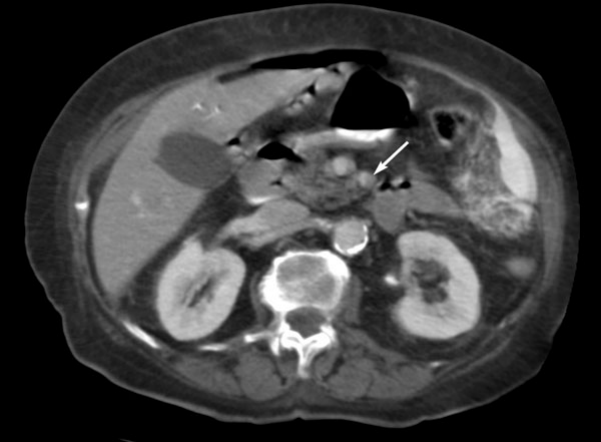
Contrast-enhanced spiral CT at a more caudal level than on Figure 2 demonstrates significant stenosis of the proximal part of the superior mesenteric artery (arrow).
